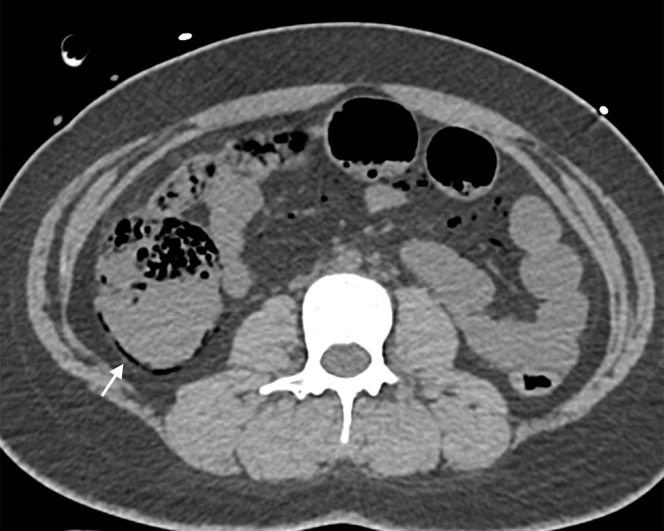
Axial abdominal computed tomography: colonic pneumatosis intestinalis (arrow, ascending colon) was the critical high-risk feature—this finding, combined with porto-mesenteric venous gas and hypotension, strongly predicted bowel ischemia, prompting urgent surgical consultation.
